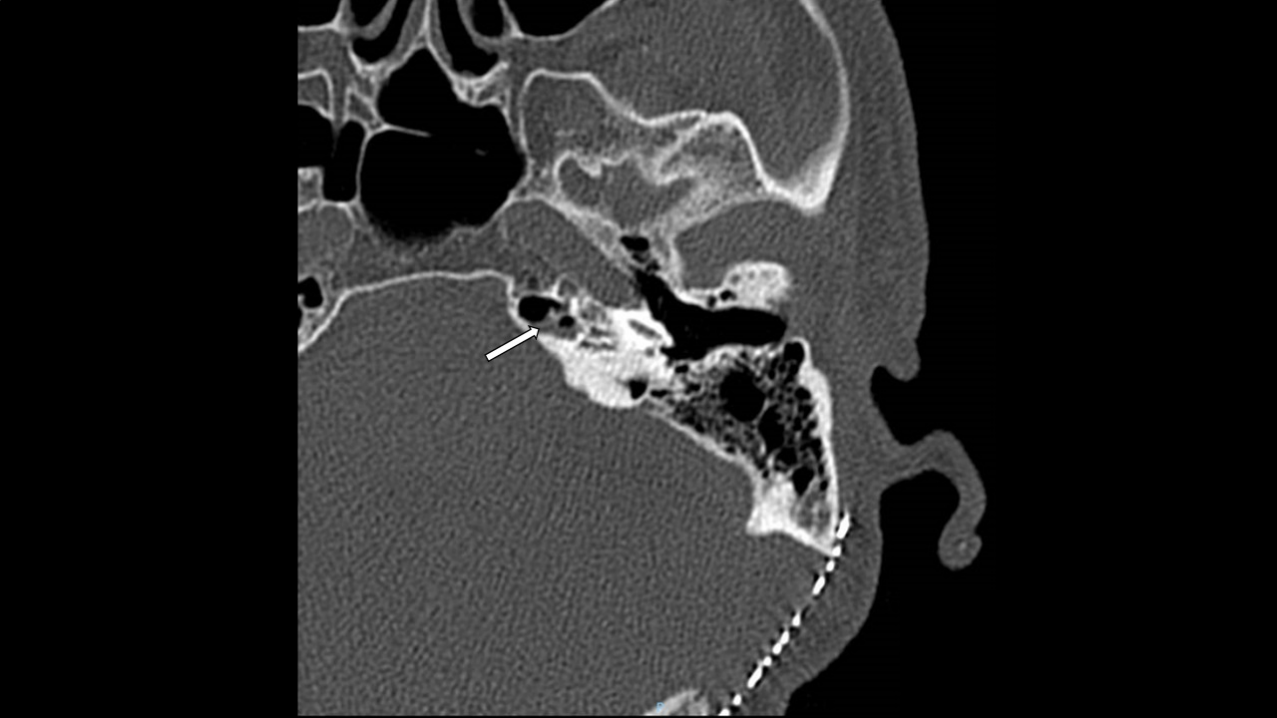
CT Temporal Bone CT temporal bone nine months after tumor resection demonstrating small air-fluid level (white arrow) in the petrous apex air cells adjacent to the petrous carotid and internal auditory canal.
